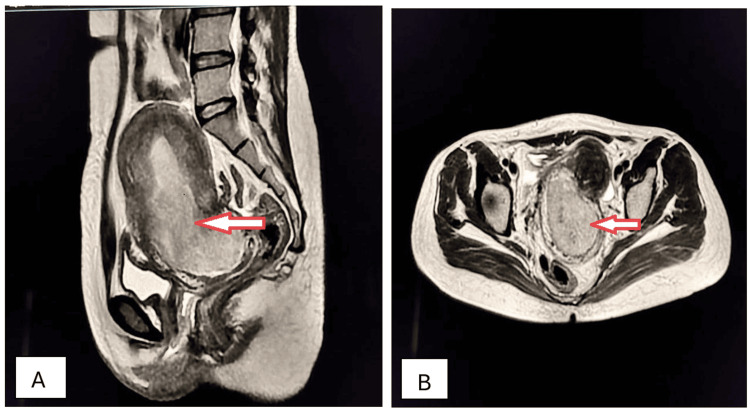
Carcinoma of endometrium with anterior and posterior wall invasion (arrows). Sagittal (A) and axial (B) T2W MRI imaging shows a well-circumscribed, inhomogeneous, predominantly hyperintense mass in the endometrial cavity and extending into the lower uterine segment and cervical canal, causing thinning of the cervical walls with anterior and posterior wall infiltration, consistent with carcinoma endometrium. T2W: T2-weighted
